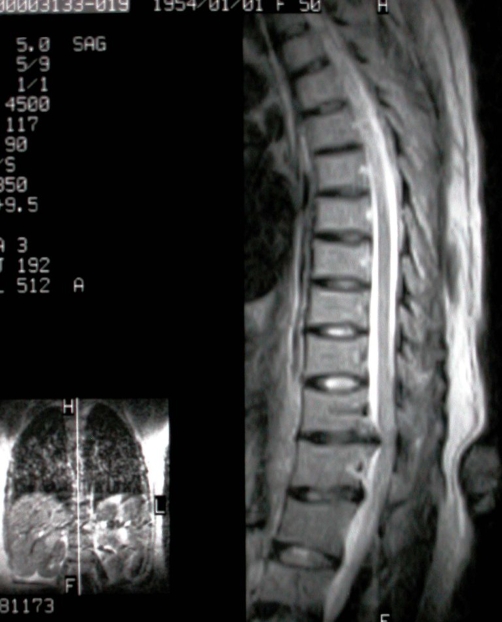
Magnetic resonance of the thoracic spine (T2-weighted, sagittal) shows disc protrusion at T11-12 level with osteophytes intruding the spinal canal.
